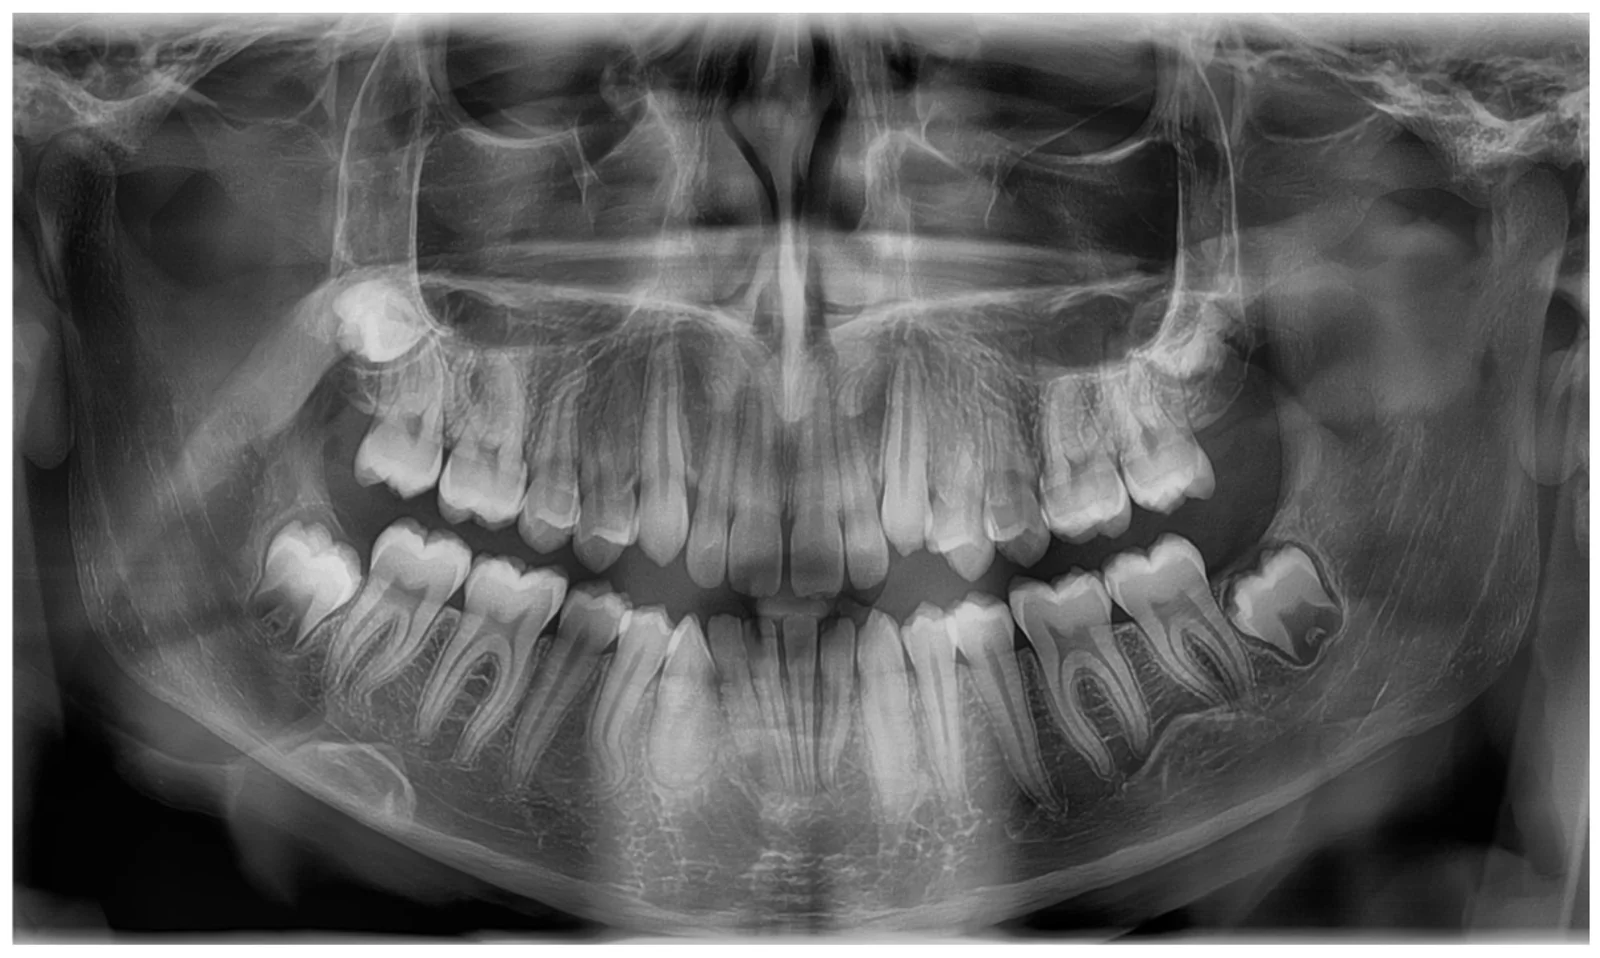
Panoramic radiograph 29 months post-surgery.
